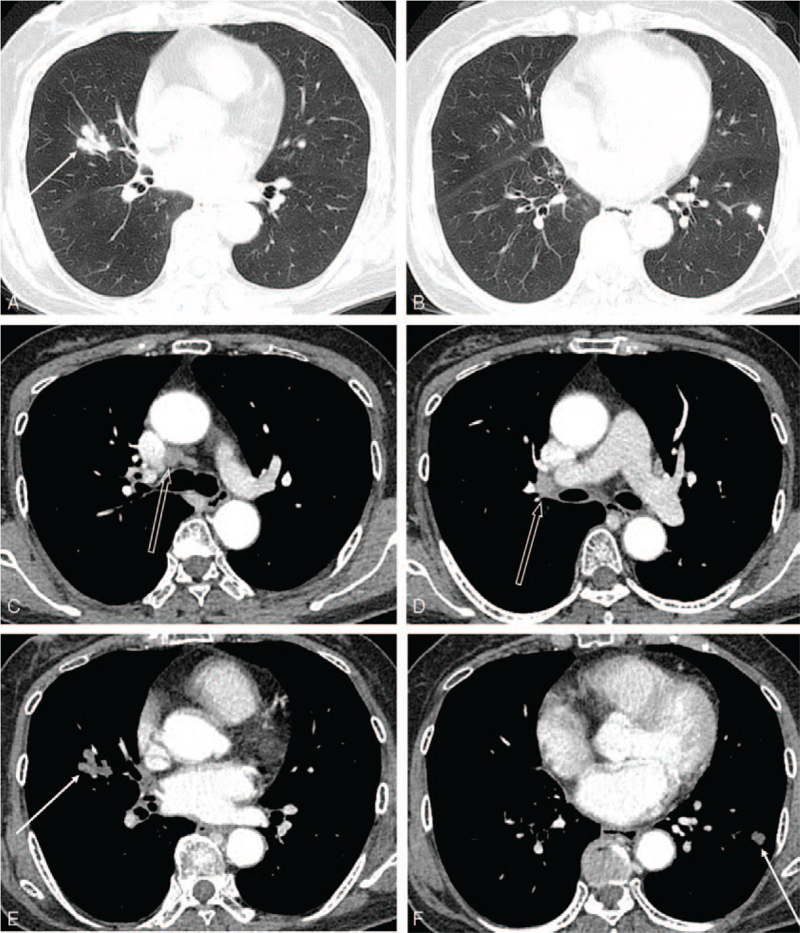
An initial contrast-enhanced chest CT performed at referring center two weeks before visiting our hospital. (A,B) On lung window setting, 2 lobulated nodules are seen in the right middle lobe and the left lower lobe (arrows). (C—F) On mediastinal window setting, there is right lower paratracheal and right hilar lymphadenopathy with homogenous enhancement without necrosis (open arrows in C,D). Contrast enhancement of the nodules in the right middle lobe and the left lower lobe is seen (arrows in E,F). The nodule in the right middle lobe shows peripheral lobulation and air-bronchogram. Another solid nodule in the left lower lobe also shows slight lobulation.
